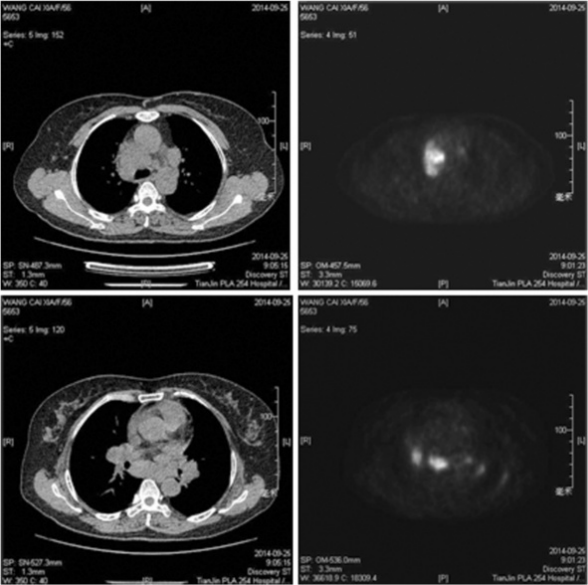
PET-CT scan. PET-CT revealed abnormal FDG uptake in the lymph nodes of the upper mediastinum and bilateral hilar areas of the lungs
